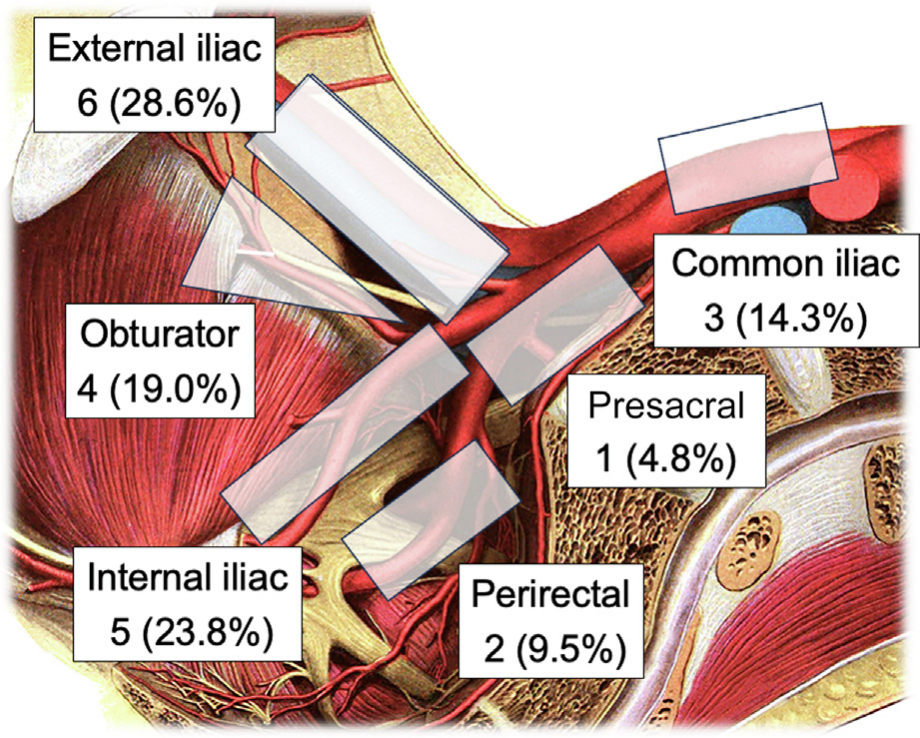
Location of node targets on preoperative PSMA PET imaging. PET = positron emission tomography; PSMA = prostate-specific membrane antigen. (License information for open access figure: “Sobotta 1909 fig.568 - Blood vessels and nervus of the pelvic wall - no labels” at AnatomyTOOL.org by Johannes Sobotta and dream_studio3, license: Creative Commons Attribution-ShareAlike.)
